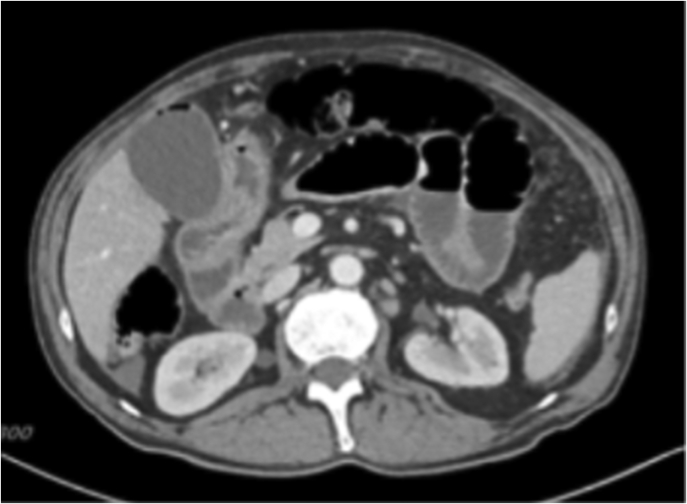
A transverse CT scan of the abdomen identifying severe enteritis and biliary gas within the gallbladder and the bile duct.
